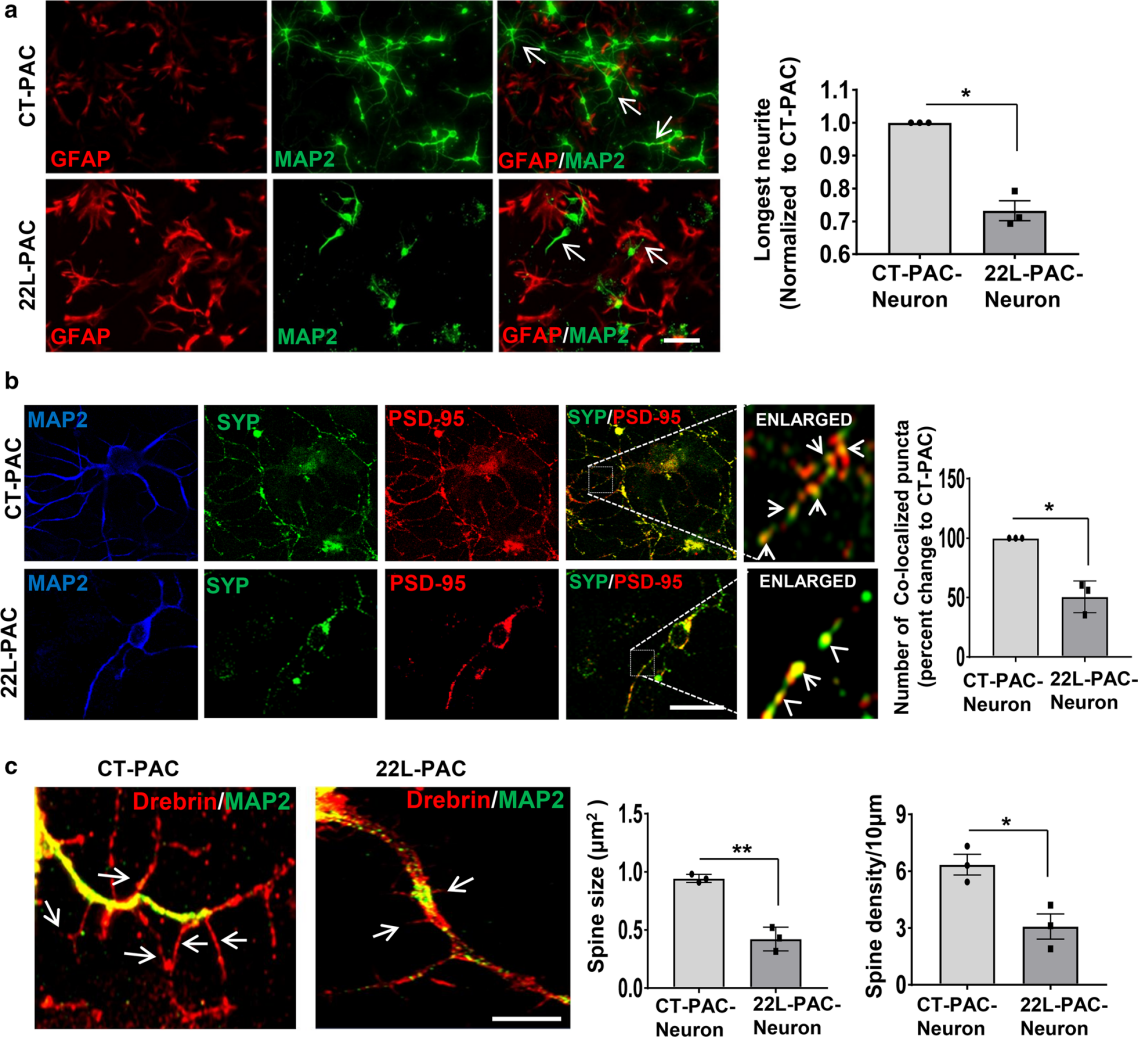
22L-derived reactive astrocytes show impairment in supporting neuronal growth. Primary cortical neurons were plated on CT-PACs or 22L-PACs and co-cultured for 10–12 days*.*
